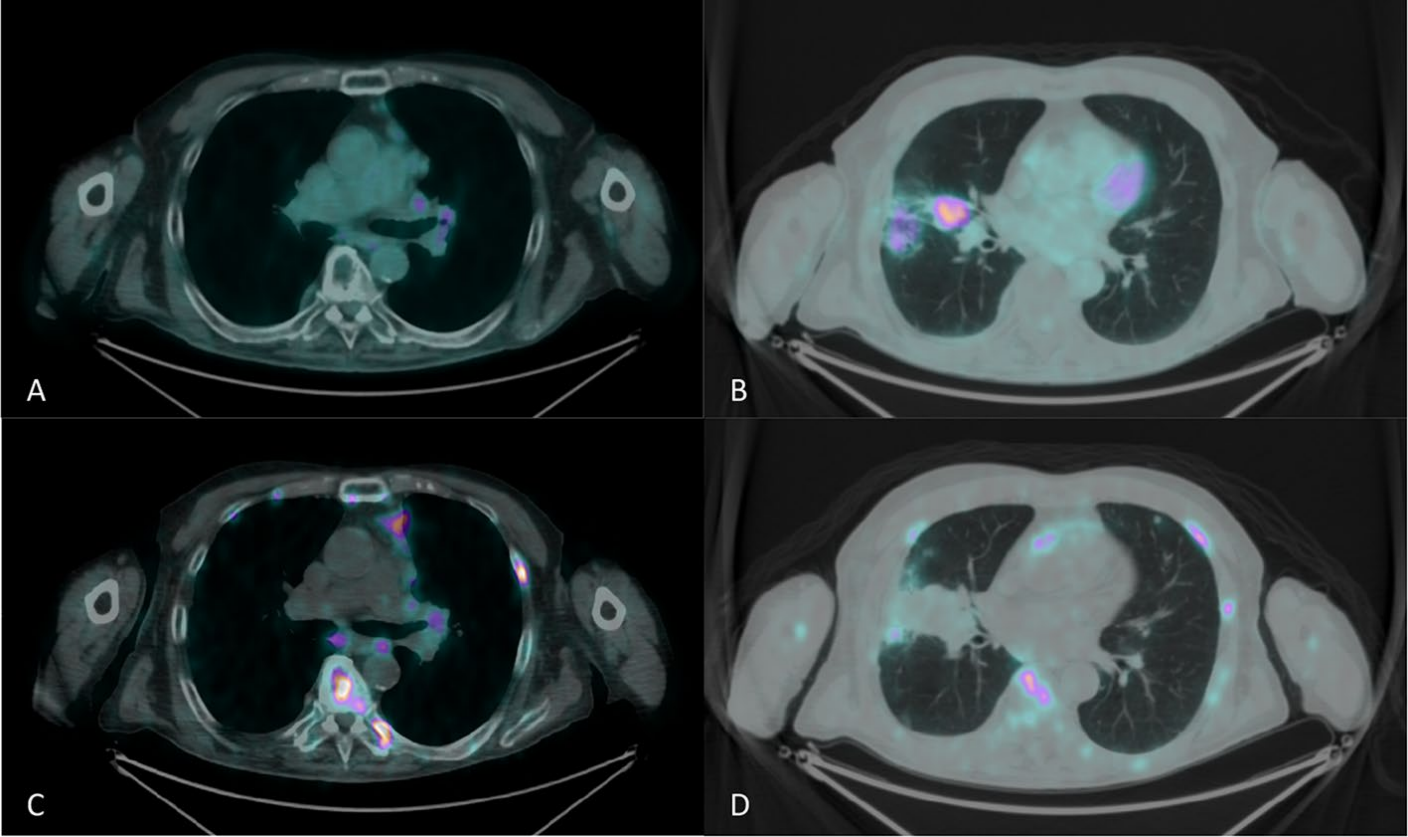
[^18^F]FDG PET/CT showed increased uptake in mediastinal lymph nodes (a) and post-actinic changes in the middle pulmonary lobe (b). [^68^Ga]Ga-DOTA-TOC PET/CT confirmed pathological mediastinal lymph nodes (c), while showing no significant uptake in the post-actinic alterations of the middle lobe (d)
